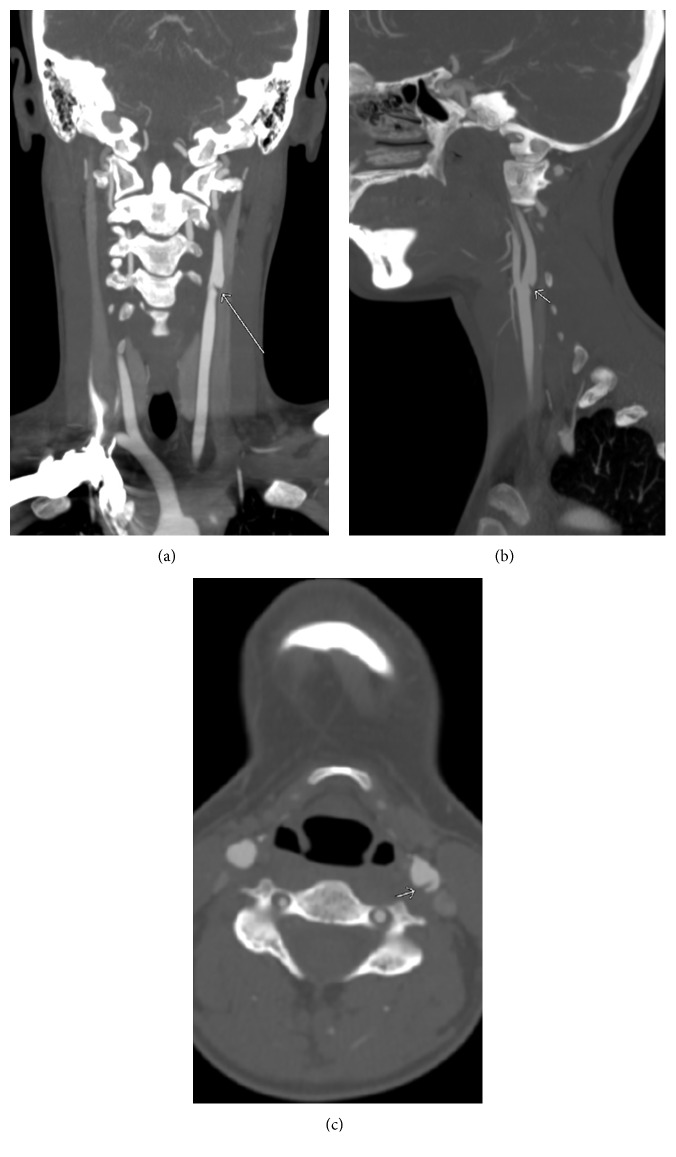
CTA neck showing carotid web in the left internal carotid artery (arrows).
